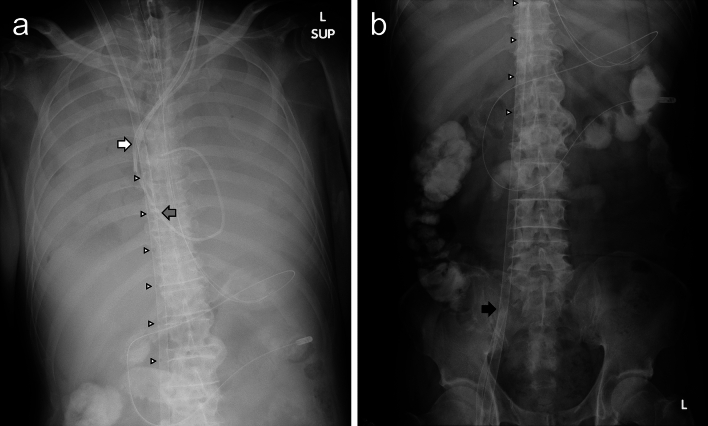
X-ray images after induction of veno-arteriovenous (V–AV) ECMO and lung rest ventilator setting. White arrow: the tip of the drainage cannula. Gray arrow: the tip of the venous reinfusion cannula. Black arrow: the tip of the arterial reinfusion cannula. White triangles: side holes of the drainage cannula. a Chest X-ray image. A venous reinfusion cannula and a central venous catheter were inserted from the right internal jugular vein. A pulmonary artery catheter and a double lumen catheter for blood purification were inserted from the left internal jugular vein. b Abdominal X-ray image. A drainage cannula was inserted from the right femoral vein and an arterial reinfusion cannula was inserted from the right femoral artery
